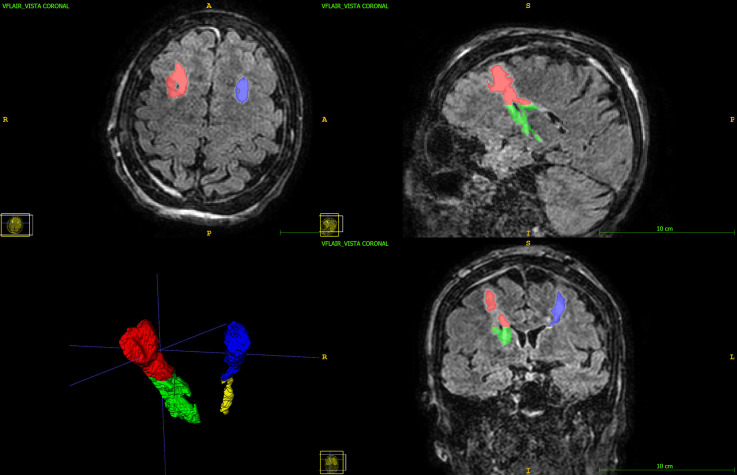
3D FLAIR image with multiplanar reconstruction in axial, coronal, and sagittal planes. The subcortical edema (red and blue) and the basal edema (green and yellow) Volumes of Interest are displayed.
